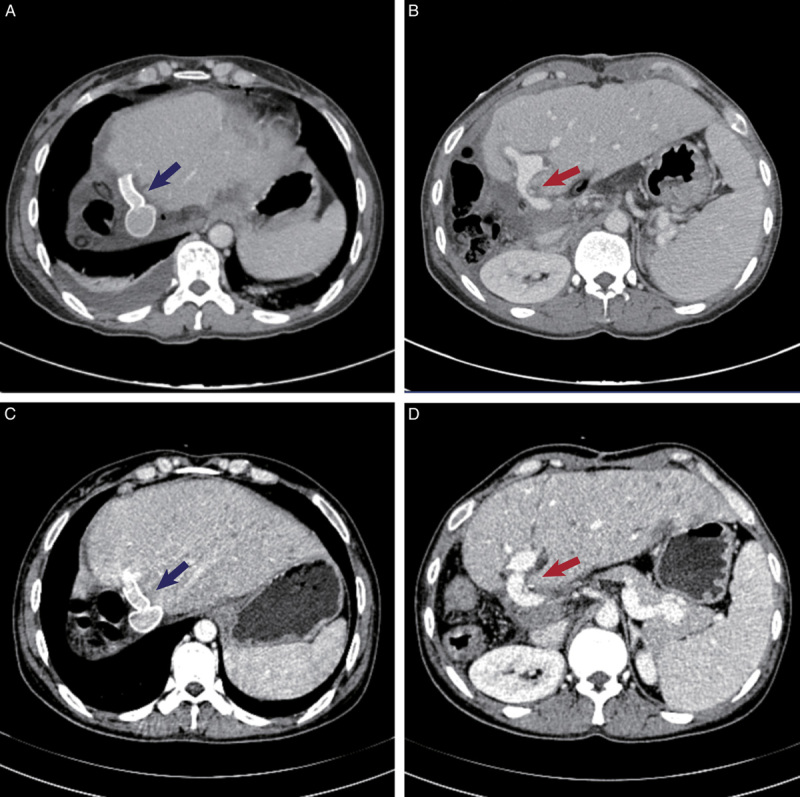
The postoperative results of CT scan. (A), (B), (C), and (D) The CT scan of Patient 3, 1 month and 1 year after surgery, respectively. As demonstrated, regenerated liver with patent reconstructed vessels and no HAE recurrence. The inferior vena cava and left hepatic vein reconstructed by artificial vascular grafts (blue arrow), portal veins (red arrow). CT, computed tomography.
